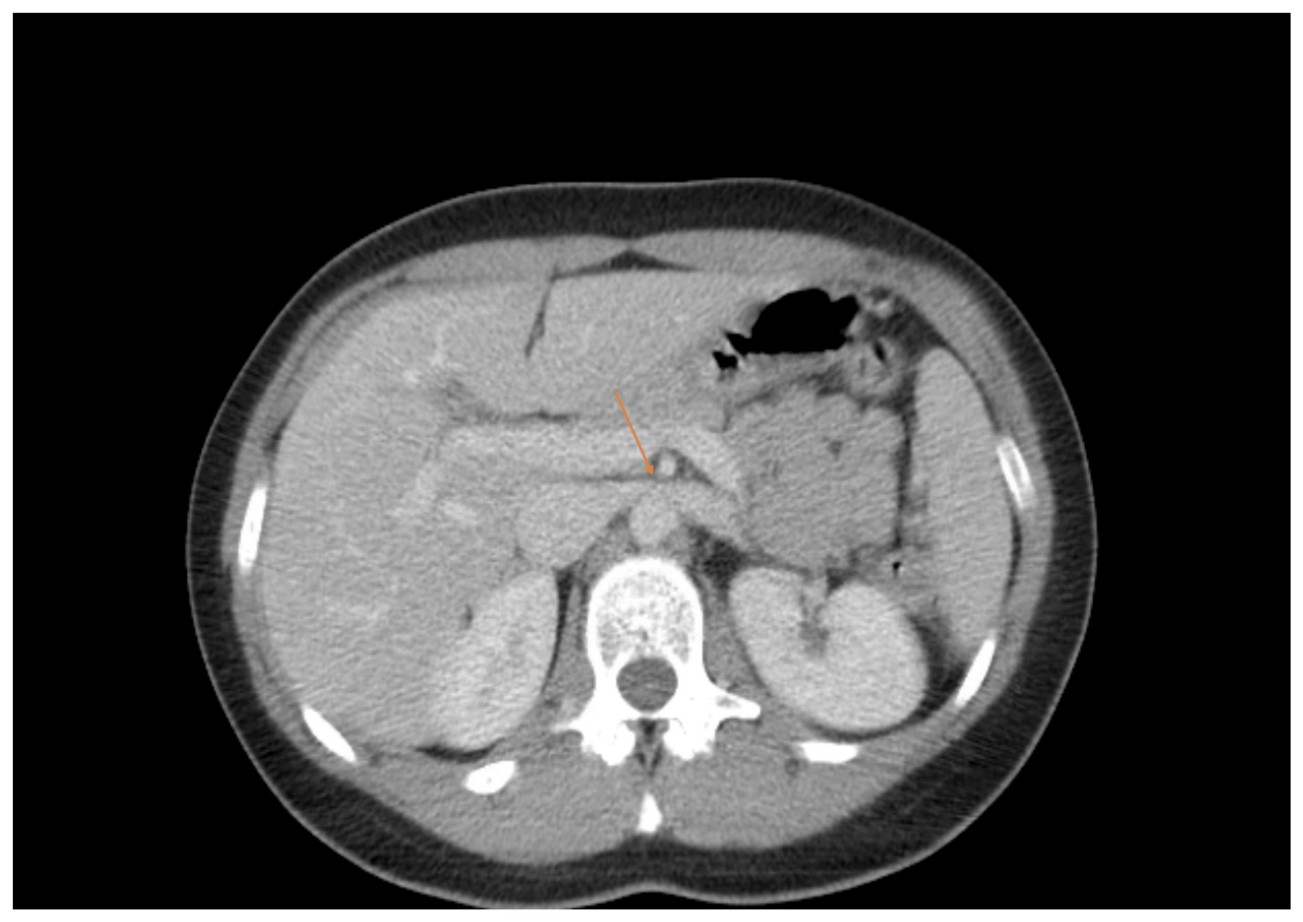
CT showing compressed LRV. CT, computed tomography; LRV, left renal vein
